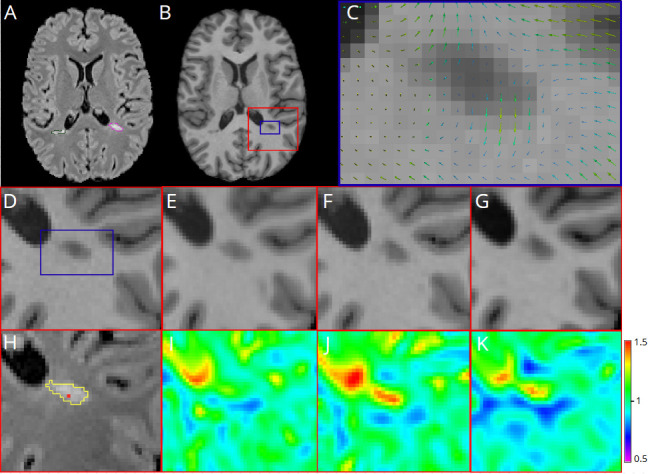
MRI-defined slowly expanding lesions (SELs) based on longitudinal deformation analysis. (A) FLAIR MRI axial slice and T2 lesions contoured at baseline, (B) T1-weighted MRI axial slice at baseline, and (C) deformation vector field from baseline to 36 months and baseline T1-weighted MRI axial slice in a region of interest corresponding to the blue box. A region of interest corresponding to the red box, with an MS lesion at its center, (D) T1-weighted MRI axial slice at baseline, (E) 12 months, (F) 24 months, and (G) 36 months. (H) FLAIR acquisition at baseline with an SEL contour in yellow and its core in red. Jacobian determinant maps in the region of interest representing relative volume growth at (I) 12 months, (J) 24 months, and (K) 36 months. Pixels in green to red (J>1
